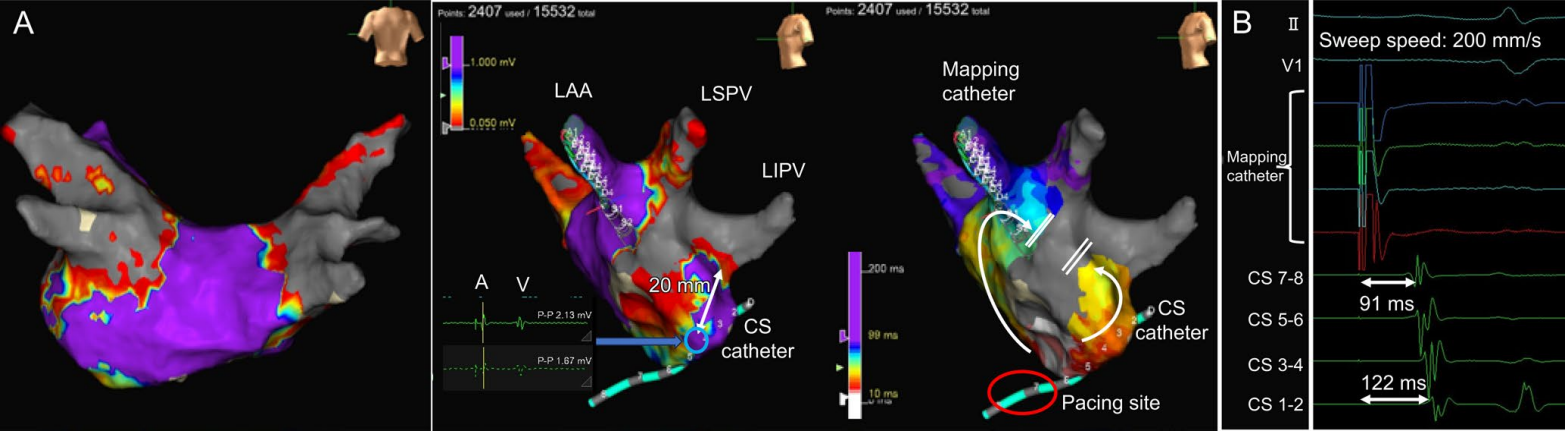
Post‐ablation mapping and intracardiac echocardiogram. (A) Post‐ablation mapping during CS ostium pacing shows a complete mitral isthmus block line. Left panel: voltage map (posterior–anterior view); middle panel: voltage map (left anterior oblique view); right panel: activation map (left anterior oblique view). (B) Pacing from the mapping catheter located in the LAA shows a proximal‐to‐distal activation pattern in the CS. LSPV, left superior pulmonary vein; LIPV, left inferior pulmonary vein; LAA, left atrial appendage; CS, coronary sinus.
